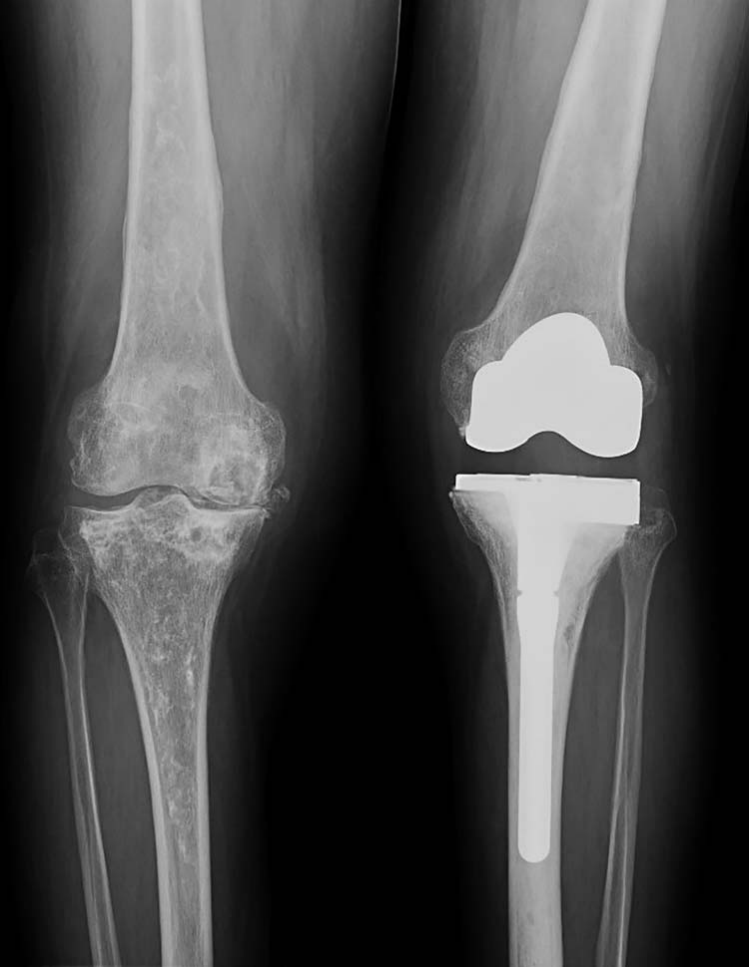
Evolution of the right knee by standing radiographs during follow-up with progression toward joint space disappearance (Kellgren–Lawrence 4) in a severe varus deformity.
